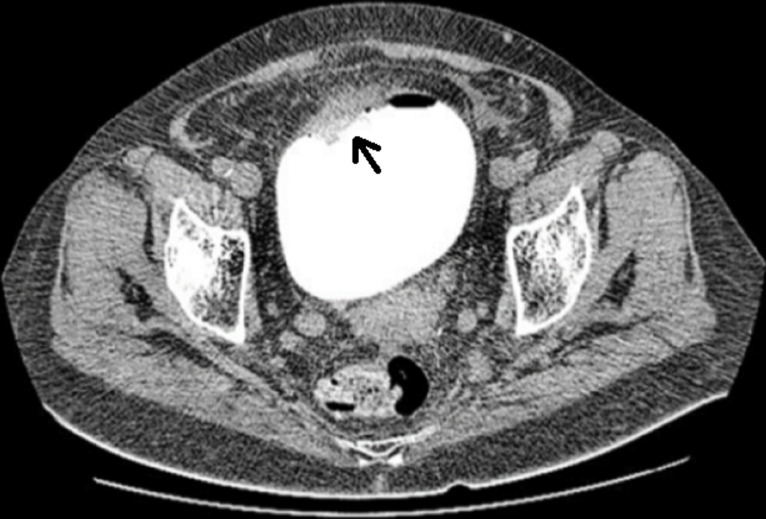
abdominal computed tomography scan with retrograde contrast showing the tumour mass (arrow)
